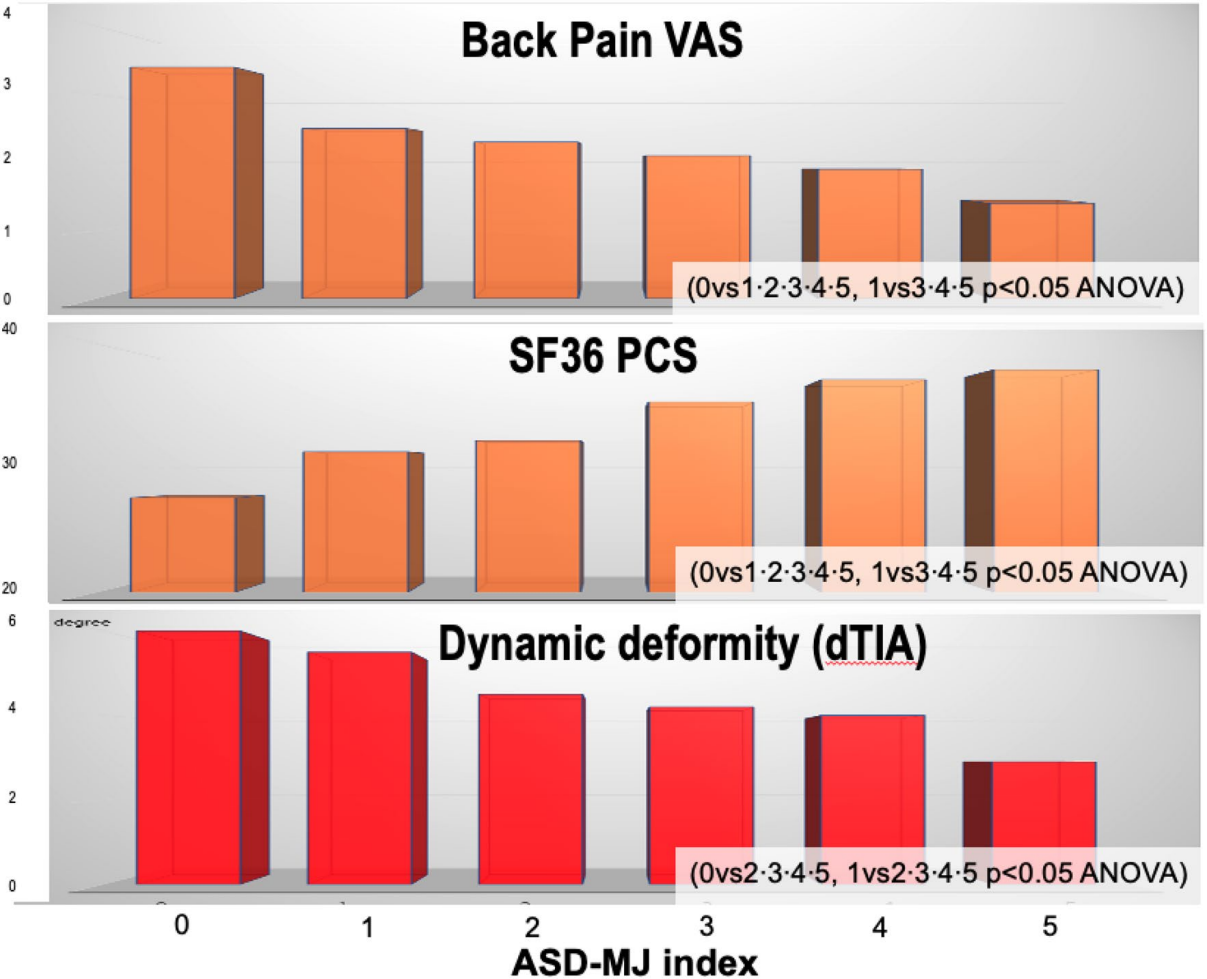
ASD-MJ & Non-radiographic parameters. VAS: visual analogue scale. SF36 PCS: physical component scale. dTIA: difference in trunk inclination angle. ASD-MJ: adult spinal deformity muscle and joint factor. ANOVA analysis of variance.
